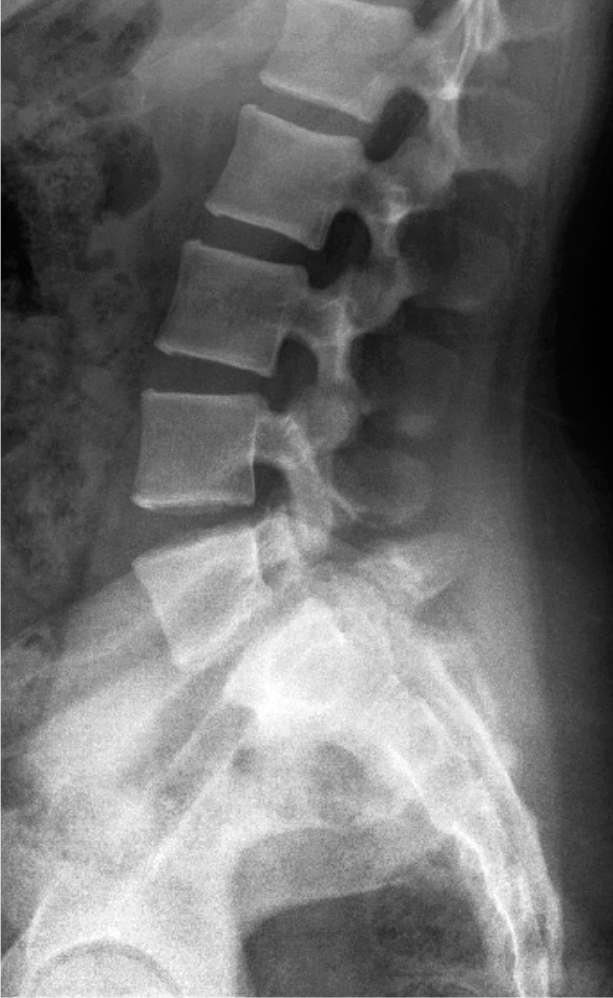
Low-grade spondylolisthesis at 2-year follow-up radiograph after non-union of bilateral L5 spondylolysis.
